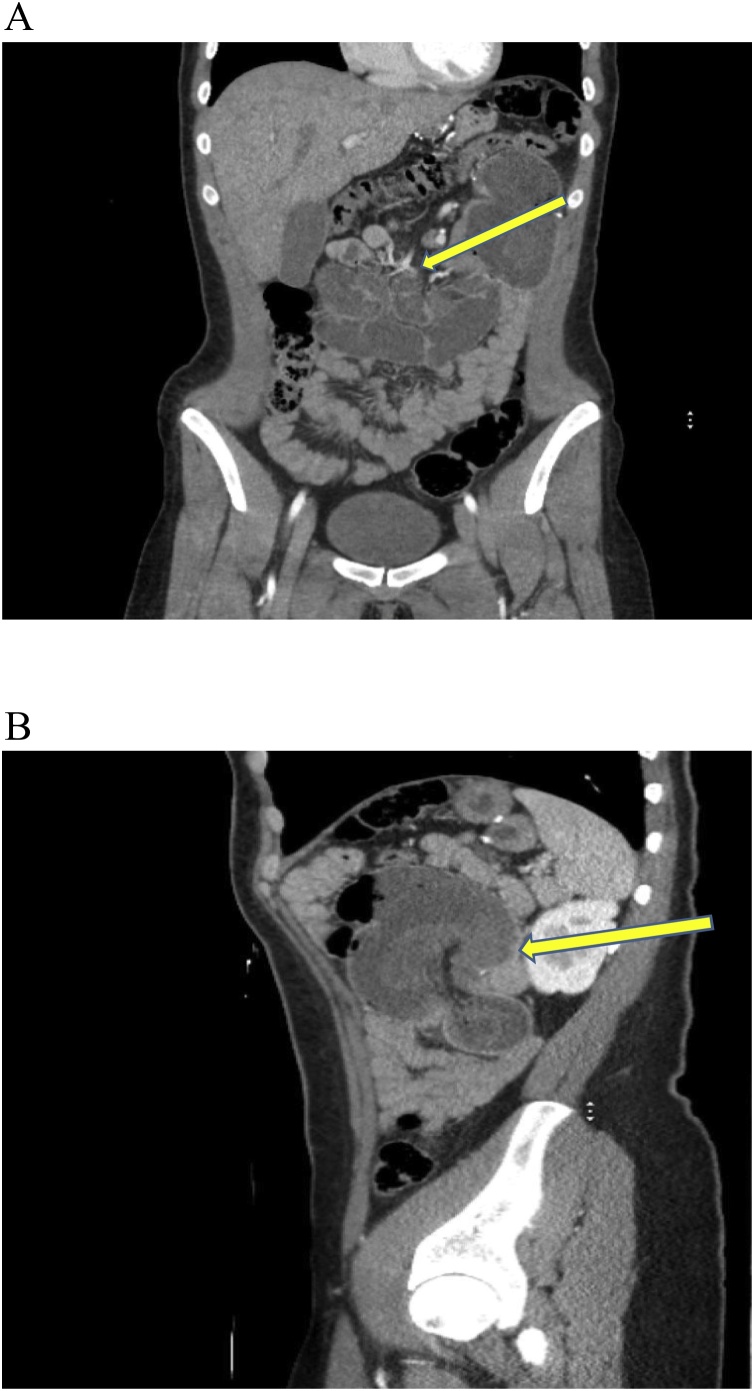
A. Coronal view of CT scan abdomen/pelvis showing a closed loop obstruction. in the left upper quadrant. Proximal to the obstruction, distended and dilated loops of bowel are seen with decompressed distal bowel after the obstruction site at the J-J anastomosis. The left upper quadrant mass is causing the obstruction at the J-J anastomotic site. Fig. 1B. Sagittal view of CT scan abdomen/pelvis showing large (grapefruit size) mass at the left upper quadrant caused by closed loop small bowel obstruction. This view shows the internal hernia where the loop of bowel herniated through the mesenteric defect at the meso-jejunal defect underneath the *J*-J anastomosis.
